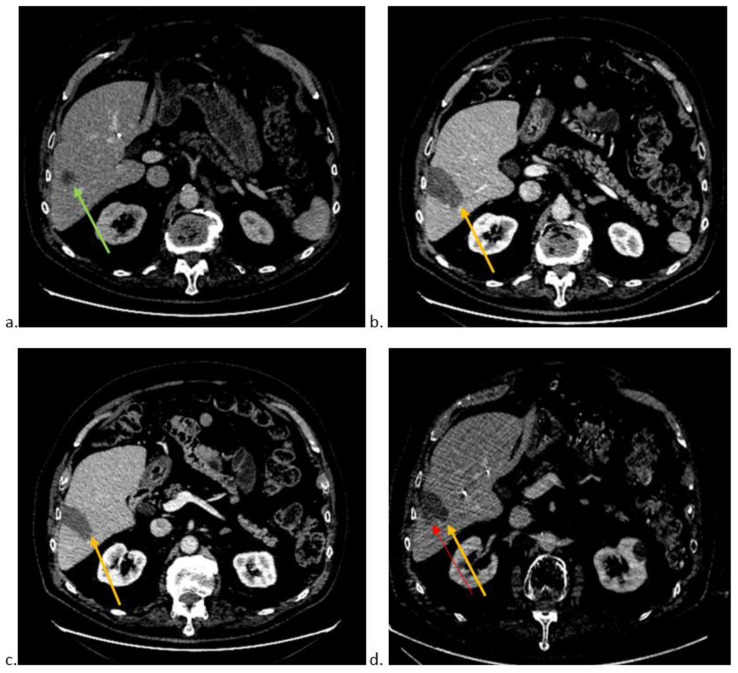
(a). Axial CT-scan image at portal phase showing colorectal liver metastasis (green arrow) in segment VI. (b). Axial CT-scan image at portal phase showing technical success of thermoablation (orange arrow) with no residual tumour. (c). Axial CT-scan image at portal phase of the lowest part of thermoablation area (orange arrow). (d). Axial CT-scan image at portal phase showing local recurrence (red arrow) at the edge of the thermoablation site (orange arrow).
